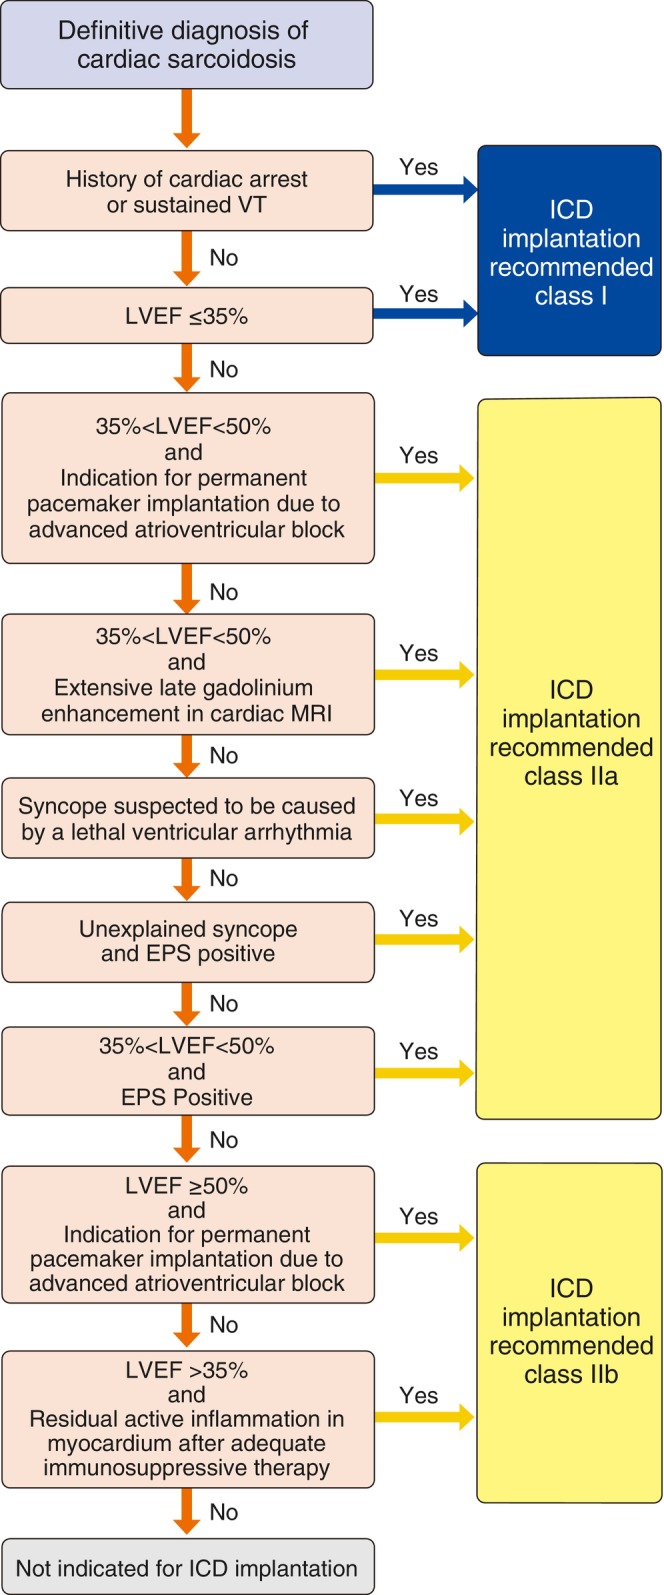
Algorithm for ICD implantation. CMR, cardiac magnetic resonance imaging; EPS, electrophysiological study; ICD, implantable cardioverter defibrillator; LVEF, left ventricular ejection fraction; VF, ventricular fibrillation; VT, ventricular tachycardia.
